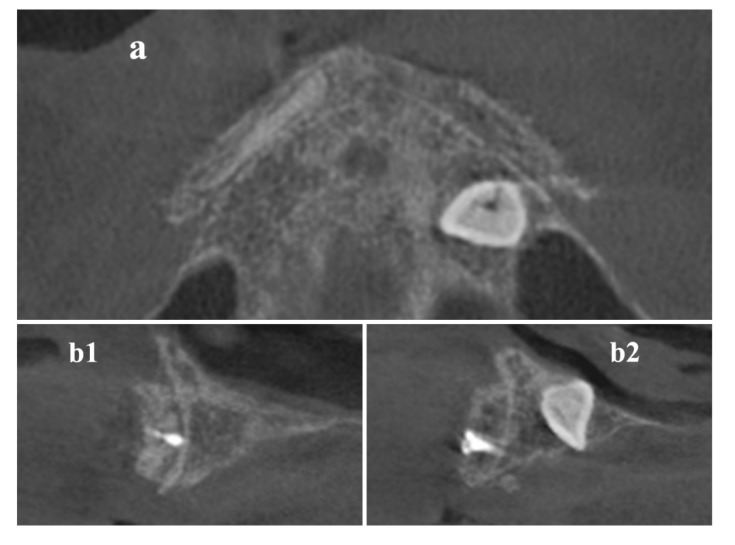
CBCT scans were done after 9 months to define implant placement width and length, on (a) axial and (b1,b2) cross-sectional images.
